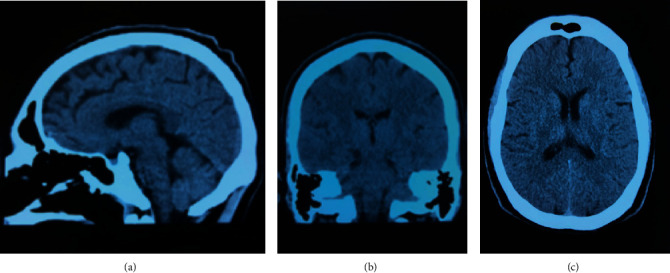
Noncontrast computed tomography of the head.
